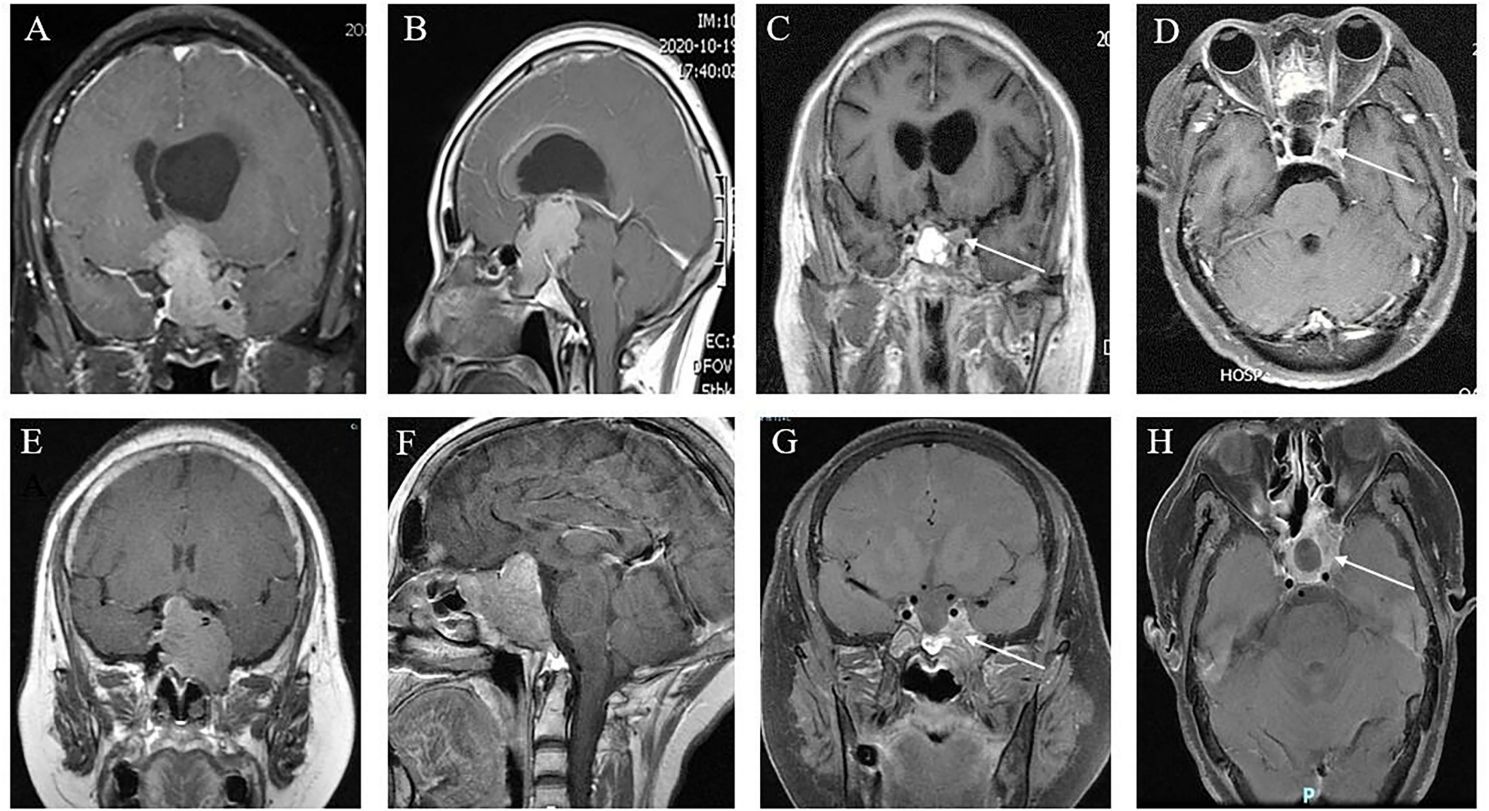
Cavernous sinus invasion limits the GTR of GPA. (A, B) Preoperative coronal (A) and sagittal views (B) showing a nonfunctional GPA with significant invasion of the sphenoid sinus and left cavernous sinus, encasement of the left internal carotid artery, and compression of the third ventricle with obstructive hydrocephalus; (C, D) 6 months postoperatively, approximately 5% of tumors remained in the cavernous sinus (white arrow), which was subtotal resection. (E, F) Coronal (E) and sagittal (F) MR images of a patient with a giant GH adenoma. This tumor is also sphenoid sinus occupying, cavernous sinus invasion, and ICA surrounding. (G, H) Three months after ETS, demonstrating that approximately 25% of tumors remained (white arrow), which were partially resected.
